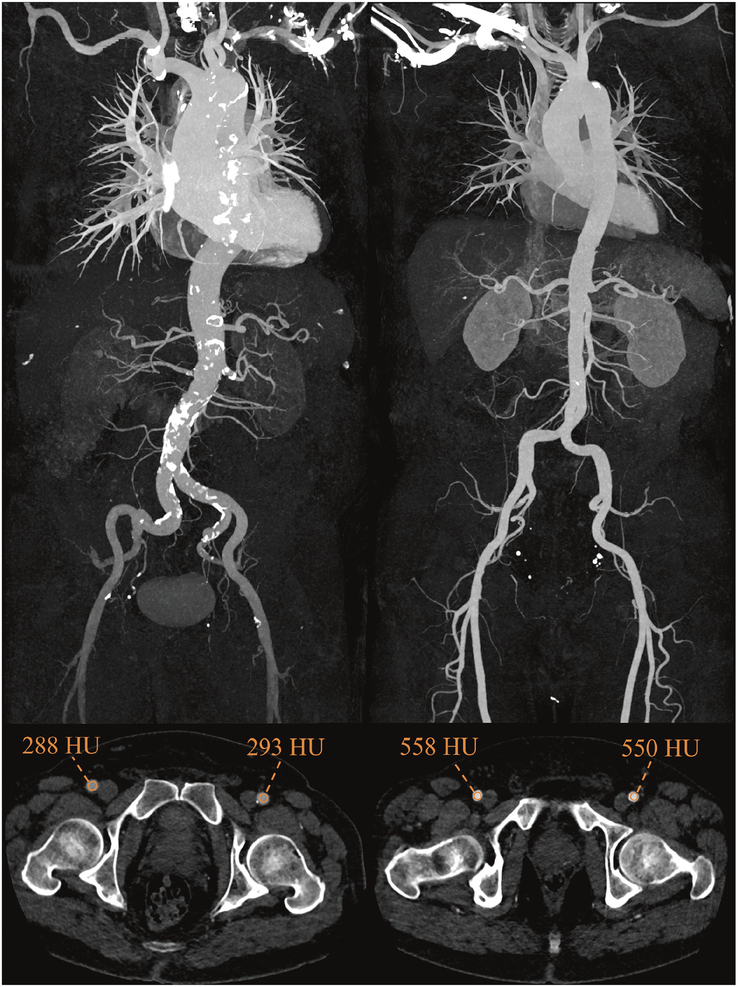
Image example—contrast attenuation along the aorta and at the femoral access site. Coronal maximum intensity projection (top row) and axial CT slice (bottom row) from representative patients imaged with a fixed trigger delay (left) and a patient-specific trigger delay (right) (window width: 800, window center: 430). Note the reduction in enhancement in the craniocaudal direction with the fixed trigger delay compared to the patient-specific trigger delay.
